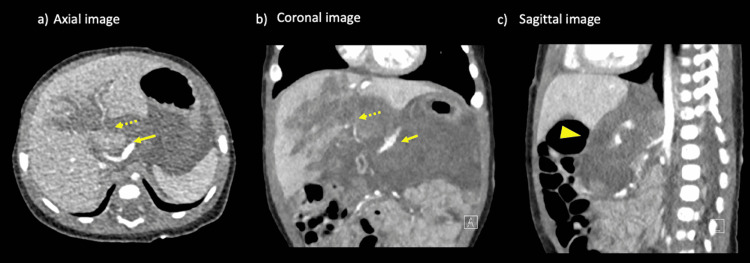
CT of the abdomen A tear in the caudate lobe to the hilar region of the liver is evident along the Cantlie line (dotted arrow). Retroperitoneal hematoma within the hepatic duodenal mesentery, from the anterior surface of the pancreas to the lesser sac (arrowhead). Pseudoaneurysm formation was observed at the site where the celiac artery transitions to the common hepatic artery (arrow).
